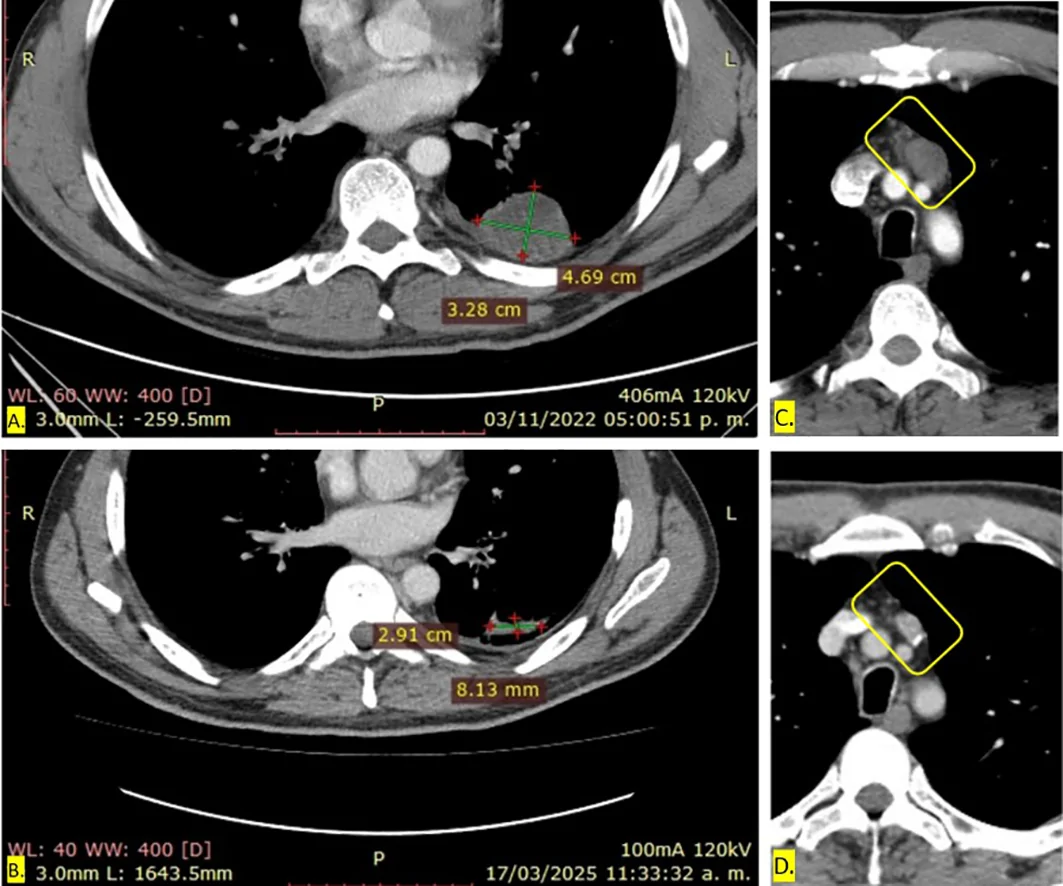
CT imaging demonstrating response to treatment in malignant melanoma. (A) Baseline (Nov 2022) contrast-enhanced CT shows a 47 × 33 mm lung mass selected as the index lesion for response assessment. (B) CT scan at 27 months (March 2025) post-treatment shows evolution to a flat, soft tissue density measuring 29×8mm, consistent with post-treatment fibrosis. (C) Baseline CT showing enlarged mediastinal lymphadenopathy (outlined in yellow). (D) CT at 27-month follow-up showing marked reduction in the previously identified lymph node (outlined in yellow) with dense, calcified appearance and peripheral calcification. The transformation from bulky, soft tissue density lymphadenopathy to small, dense, calcified residual tissue represents complete treatment response with fibrotic replacement of previously active disease.
